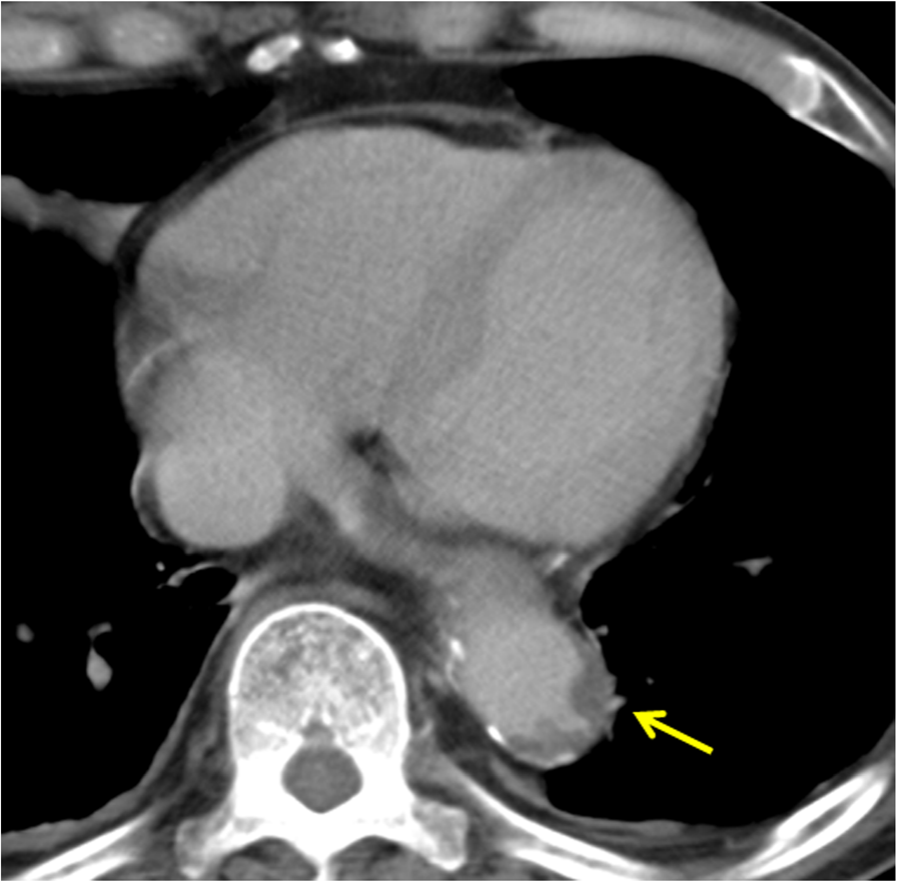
Computed tomography (CT) scan revealing shaggy aortic wall due to atherosclerosis (arrow)
